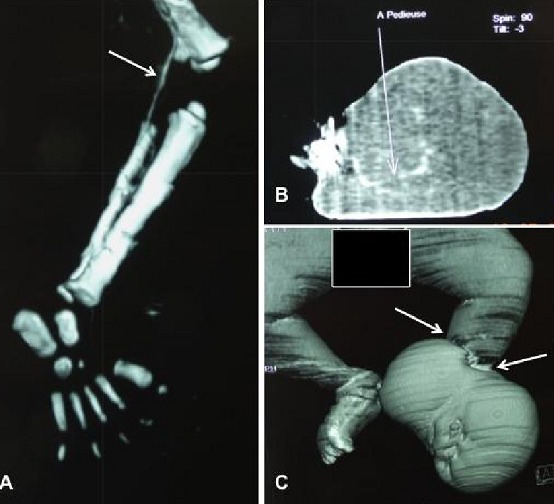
CT angiography of pelvic members in an infant of 04 months displaying amniotic bands left. A) note the left popliteal artery correctly injected, bone structures without fault; B) the vascularization is normal with a note highlighting the left pedal artery; C) necking with downstream lymphedema
